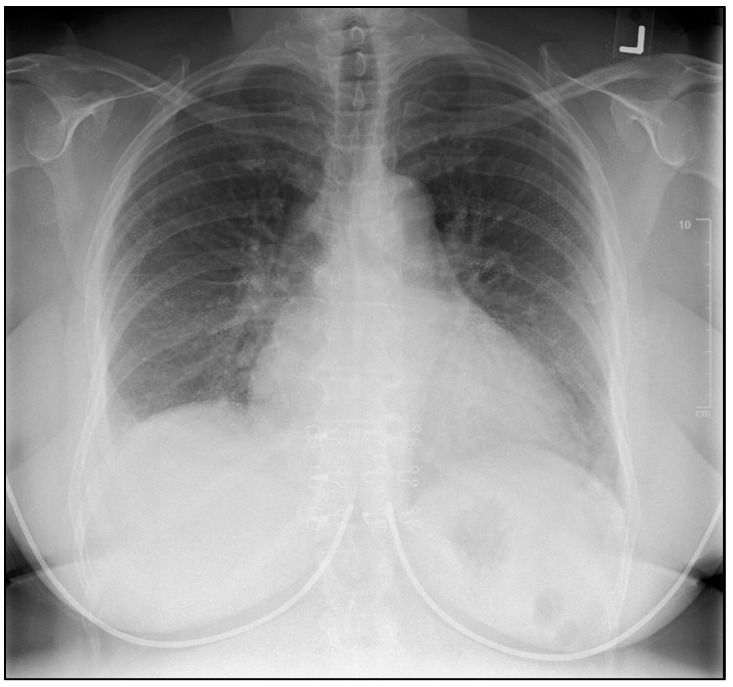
Chest X-ray demonstrating cardiomegaly and pulmonary congestion.
